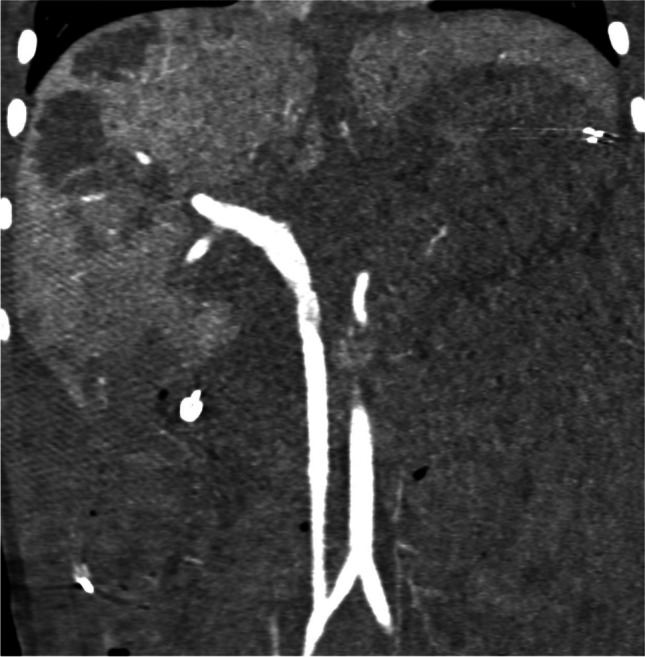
Coronal contrast-enhanced computed tomography image in a 5-year-old girl reveals contrast within a shunt graft between the right iliac artery and the portal vein to achieve portal vein arterialization in an otherwise de-arterialized liver
